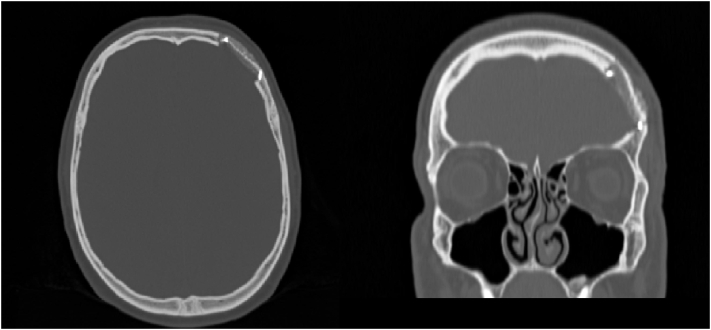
Post-operative (post-op) computing tomography (CT) scan. Left: Transversal and (right) frontal plane. Note the surgical area lacking of the hyperostotic mass.
